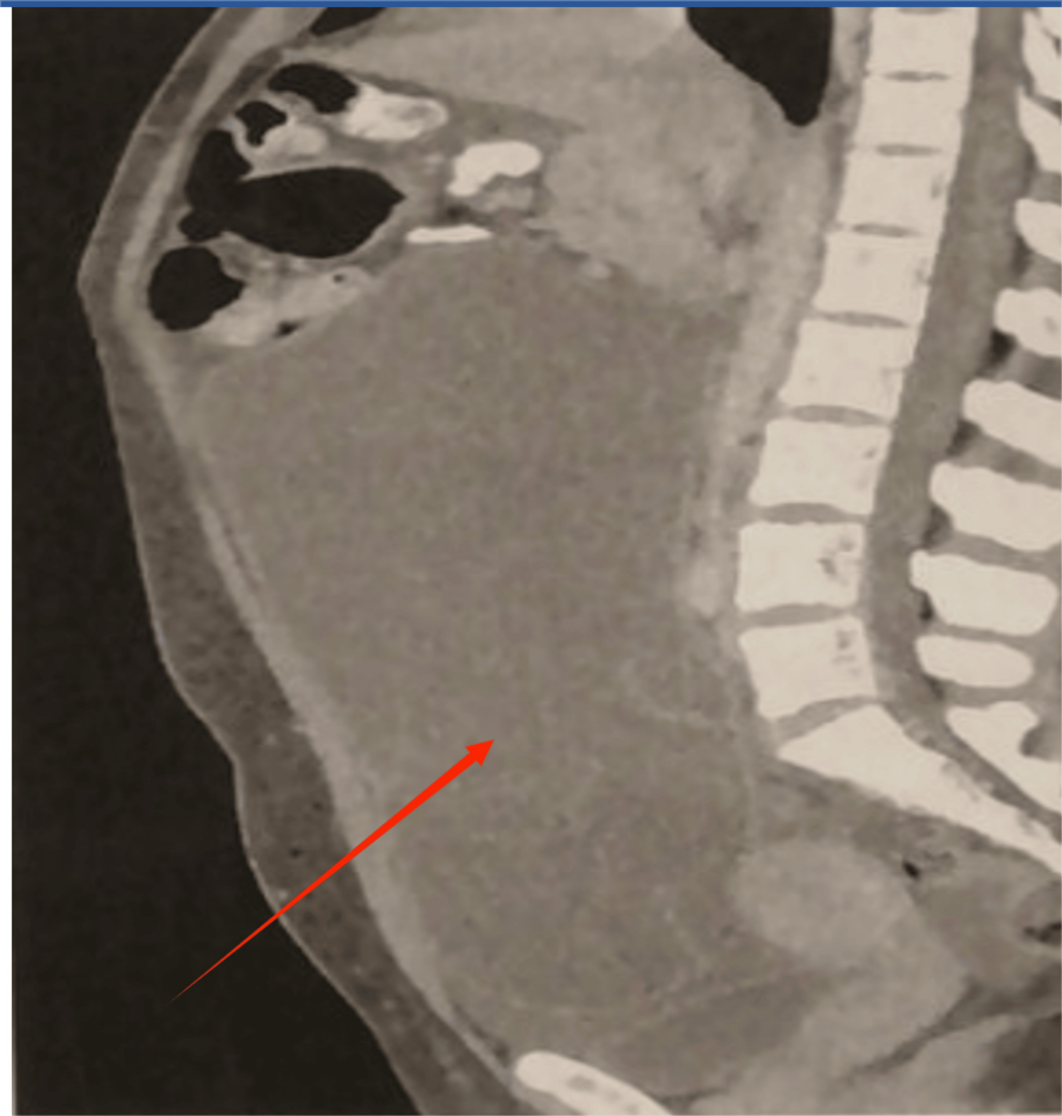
Sagittal abdominal magnetic resonance imaging (MRI) revealed a large, near-water-density cystic mass with internal thick septations and mural calcification (red arrow).
